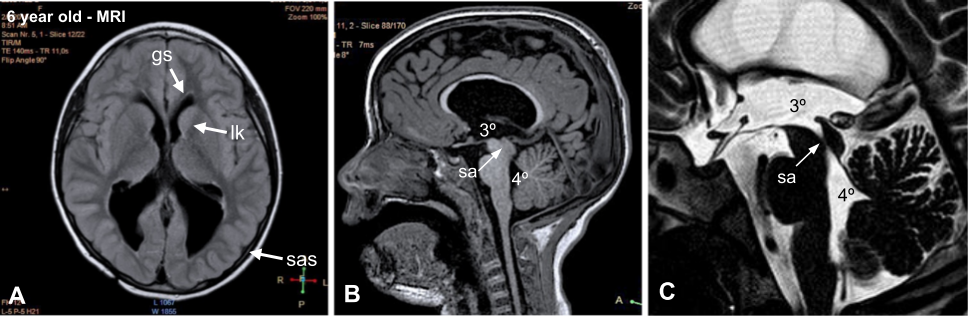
MRI findings at 6th years of age. a MRI (transverse T1 imaging) showing mild ventricular dilatation, a normal subarachnoid space (sas), signs of periventricular leukomalacia (lk) in the frontal horns of the lateral ventricles, and a glial scar (gs) in the frontal subcortical zone. b, c MRI (sagittal T1 and sagittal T2 imaging, respectively) showing complete obliteration of the SA
